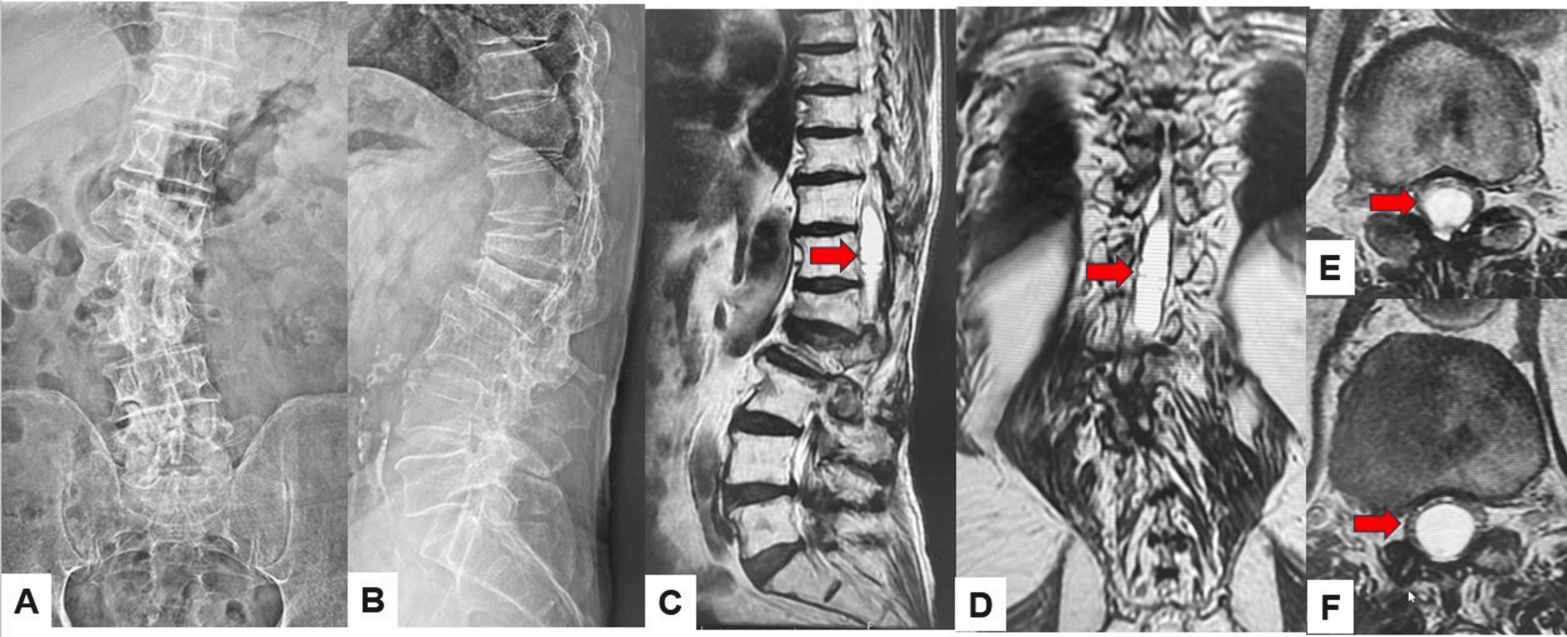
Preoperative images A: Anteroposterior lumbar radiogram, B: Lateral lumbar radiogram, C: T2-weighted mid sagittal lumbar MRI, D: T2-weighted coronal lumbar MRI, E: T2-weighted axial MRI at T12, and F: T2-weighted axial MRI at T12/L1. Red arrows indicate a large syringomyelia.
